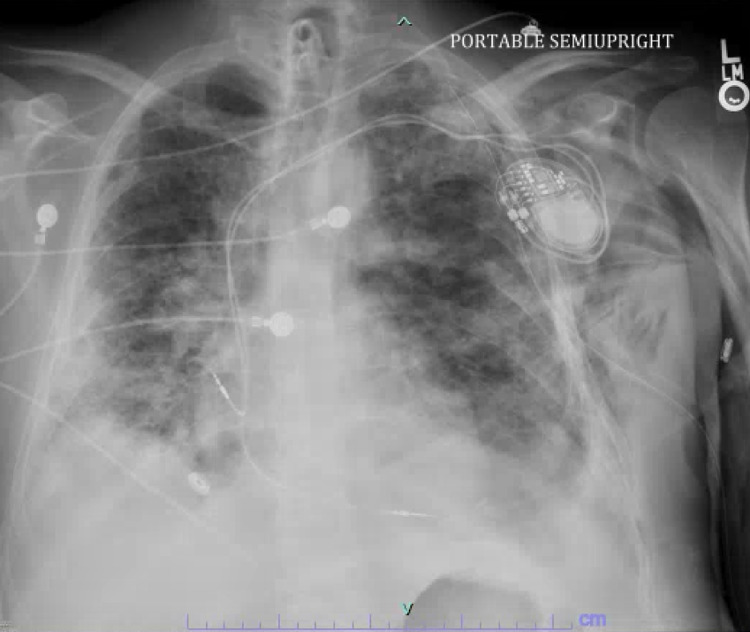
Chest X-ray after pacemaker placement
